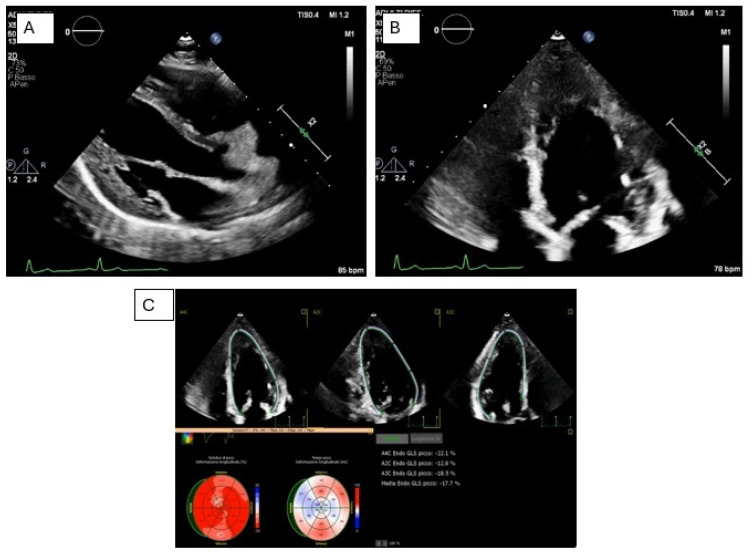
Echocardiographic findings in a young patient with Fabry disease. Panel (A). Parasternal long-axis view showing a left ventricle with normal internal dimensions and mildly increased wall thickness, predominantly involving the mid and apical inferior, inferolateral, and lateral segments (maximum wall thickness 14 mm; interventricular septum 9 mm; posterior wall 9 mm). Global systolic function is at the lower limit of normal (biplane Simpson LVEF 52%). No regional wall motion abnormalities are observed. Panel (B). Apical four-chamber view with apical zoom confirming preserved LV cavity size and mild segmental hypertrophy, particularly the apex. Panel (C). Myocardial deformation analysis demonstrating impaired longitudinal function with a reduced global longitudinal strain (GLS −16.9%).
